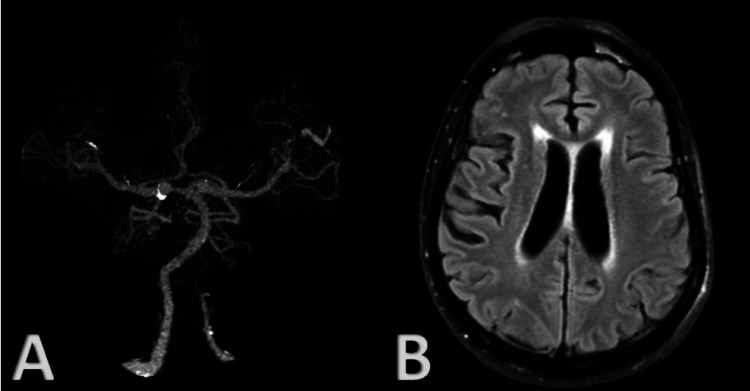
(A) 3D reconstruction of CTA head demonstrating normal intracranial anterior and posterior circulation. (B) Axial FLAIR sequence MRI brain demonstrating chronic microvascular changes particularly in the periatrial region. 3D: three-dimensional; CTA: computed tomography angiography; FLAIR: fluid-attenuated inversion recovery; MRI: magnetic resonance imaging
